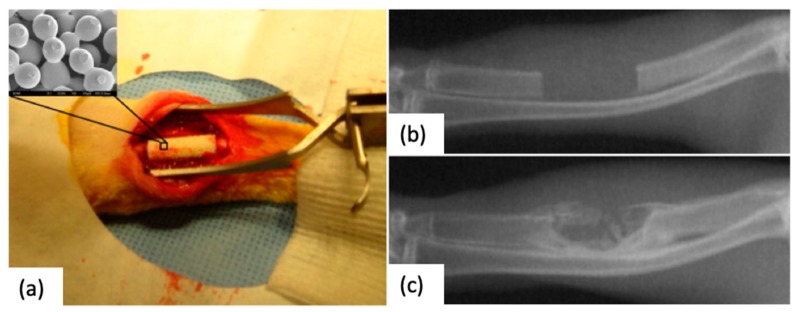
(a) Implantation of a sintered microsphere-based scaffold in a 15 mm induced ulna defect. (b) Appearance of the defect immediately after the surgical procedure, (c) radiograph on the defect site after 12-week post-operation with clearly new bone formation [43]. Figure reprinted from Acta Biomaterialia, 6, Tao Jiang, Syam P. Nukavarapu, Meng Deng, Ehsan Jabbarzadeh, Michelle D. Kofron, Stephen B. Doty, Wafa I. Abdel-Fattah, Cato T. Laurenci, Chitosan–poly(lactide-co-glycolide) microsphere-based scaffolds for bone tissue engineering: In vitro degradation and in vivo bone regeneration studies, 3457–3470, 2010, with permission from Elsevier.
